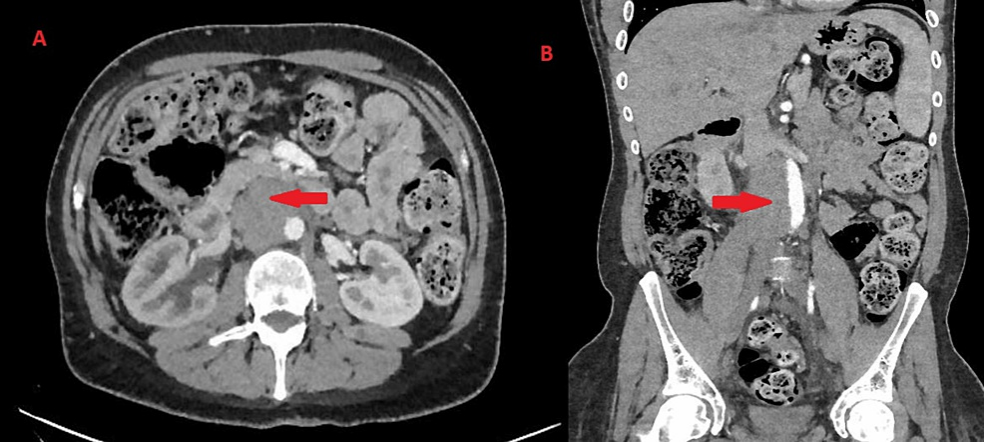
Contrast CT abdomen and pelvis Image "A" to the left is the axial view and shows para-aortic lymphadenopathy causing hydronephrosis. Image "B" to the right is the coronal view of the para-aortic nodal mass.
